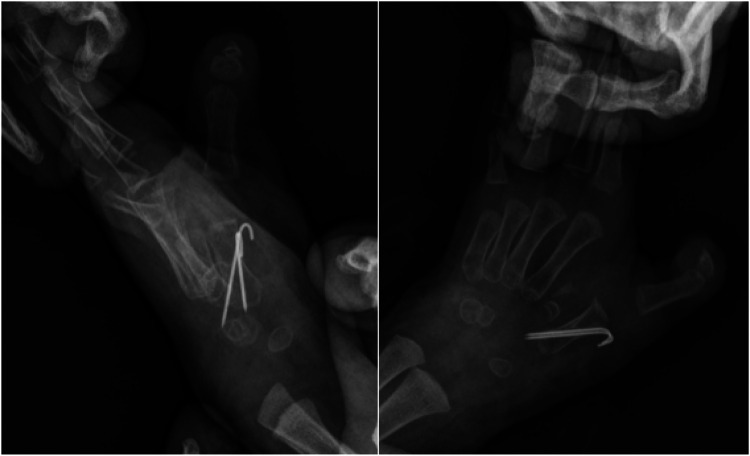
Postoperative 5-month anteroposterior and lateral radiographs of the left hand.
